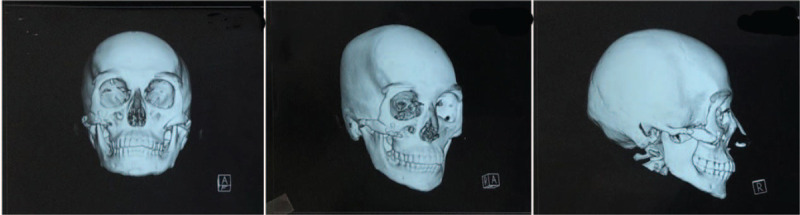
Computed tomography images of the patient showing repair of the bone defect at the 11-month review after revisional surgery. The internal fixations in the area of right zygomatic body and zygomatic arch were in place. Additionally, the bone mass was not displaced, and bone continuity in the operation area was corrected.
